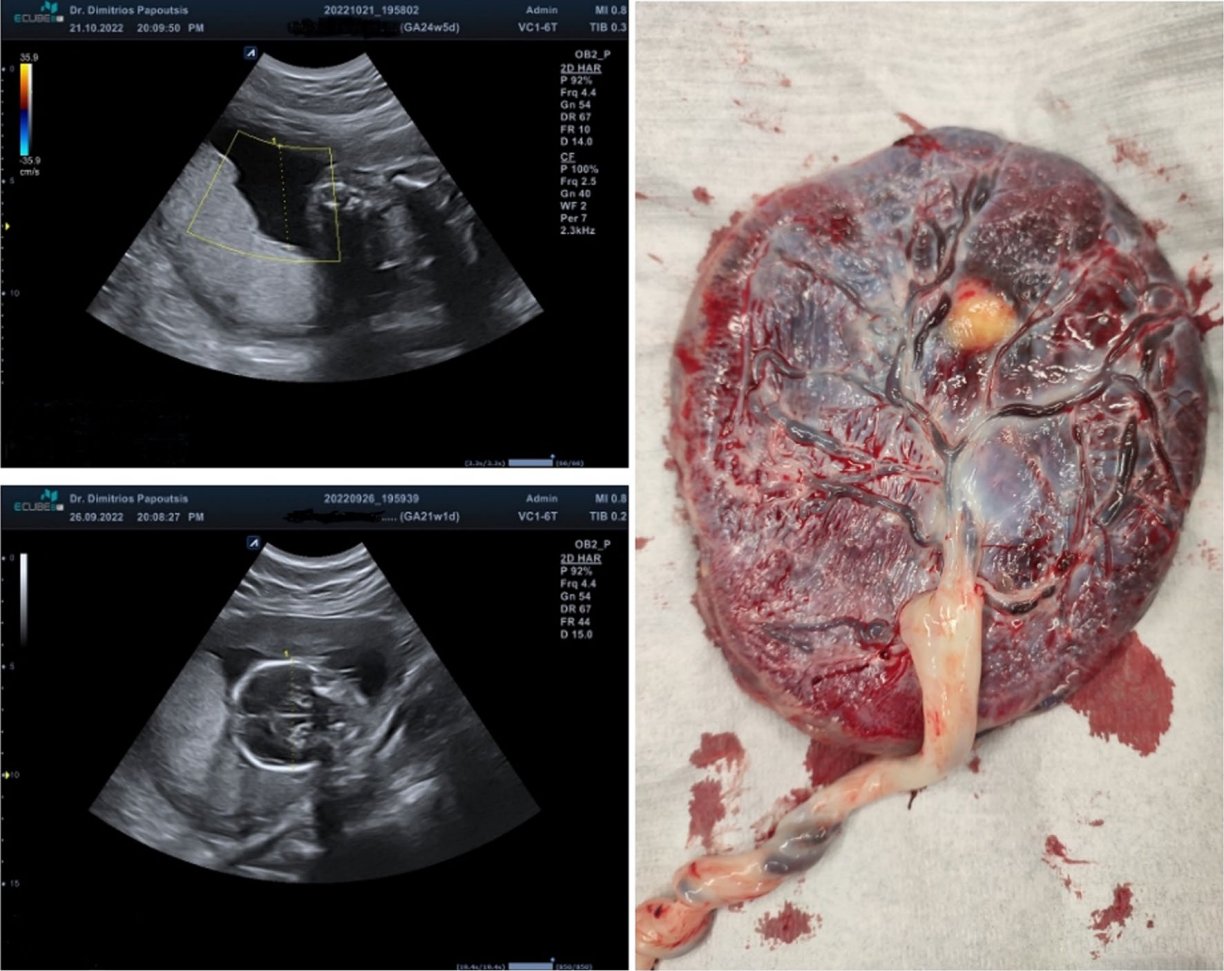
Lower left/upper left: Images of the placenta during routine ultrasound examinations at 21 and 24 weeks of gestation. There was no suspicion of any placental lesion and fetal biometry was within normal range according to gestational age. Right: The placenta in the operating room following delivery of the newborn with a well-circumscribed yellowish nodule measuring 2 × 2 cm consistent with a placental lipoma
